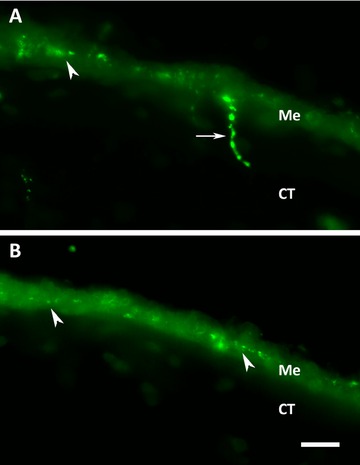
Regeneration of perpendicular fibers in the connective tissue of the intestinal rudiment. These varicose fibers (arrow) that cross from the mesothelium into the connective tissue are first observed in the proximal area of the rudiment by 14 dpe (A) using the RN1 antibody, while they are absent in the distal area of the rudiment at the same stage (B). Arrowheads signal some of the fiber varicosities in the mesothelium. Me, mesothelial layer; CT, connective tissue layer. Bar 20 μm.
